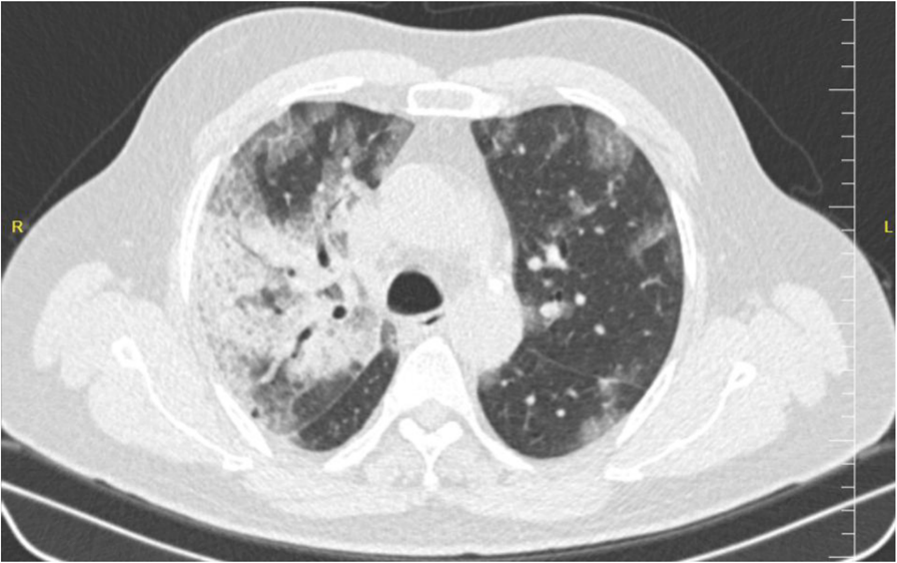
An axial chest CT from a 60-year-old man with COVİD-19 pneumonia. Non-contrast-enhanced chest CT image showed multiple peripheral ground-glass opacities distributed in the bilateral lung fields, combined with consolidation
